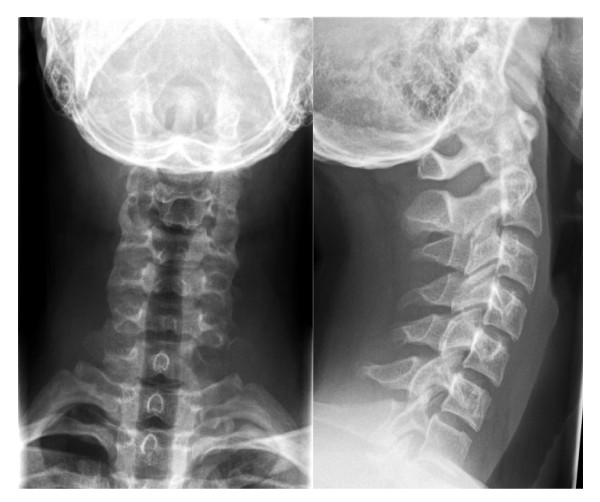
The a.p. view of the preoperative conventional X-Ray of the cervical spine shows a thinning of bone in C7 on the left.
